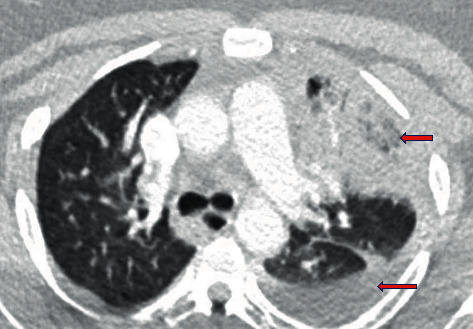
CT chest with contrast revealing extensive left upper lobe and lingular pneumonia with small lef-sided partially loculated pleural effusion.
